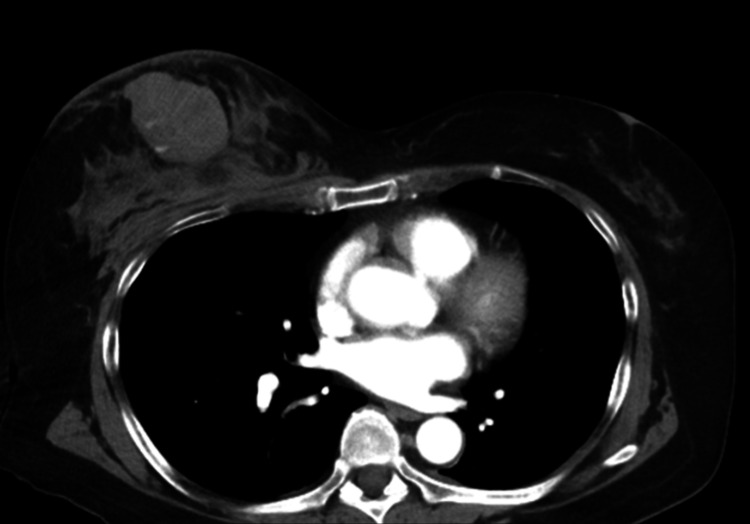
Contrast-enhanced CT Contrast-enhanced CT showing fluid collection with extravasation between the mammary gland and the pectoralis major muscle in the right anterior chest CT: computed tomography
